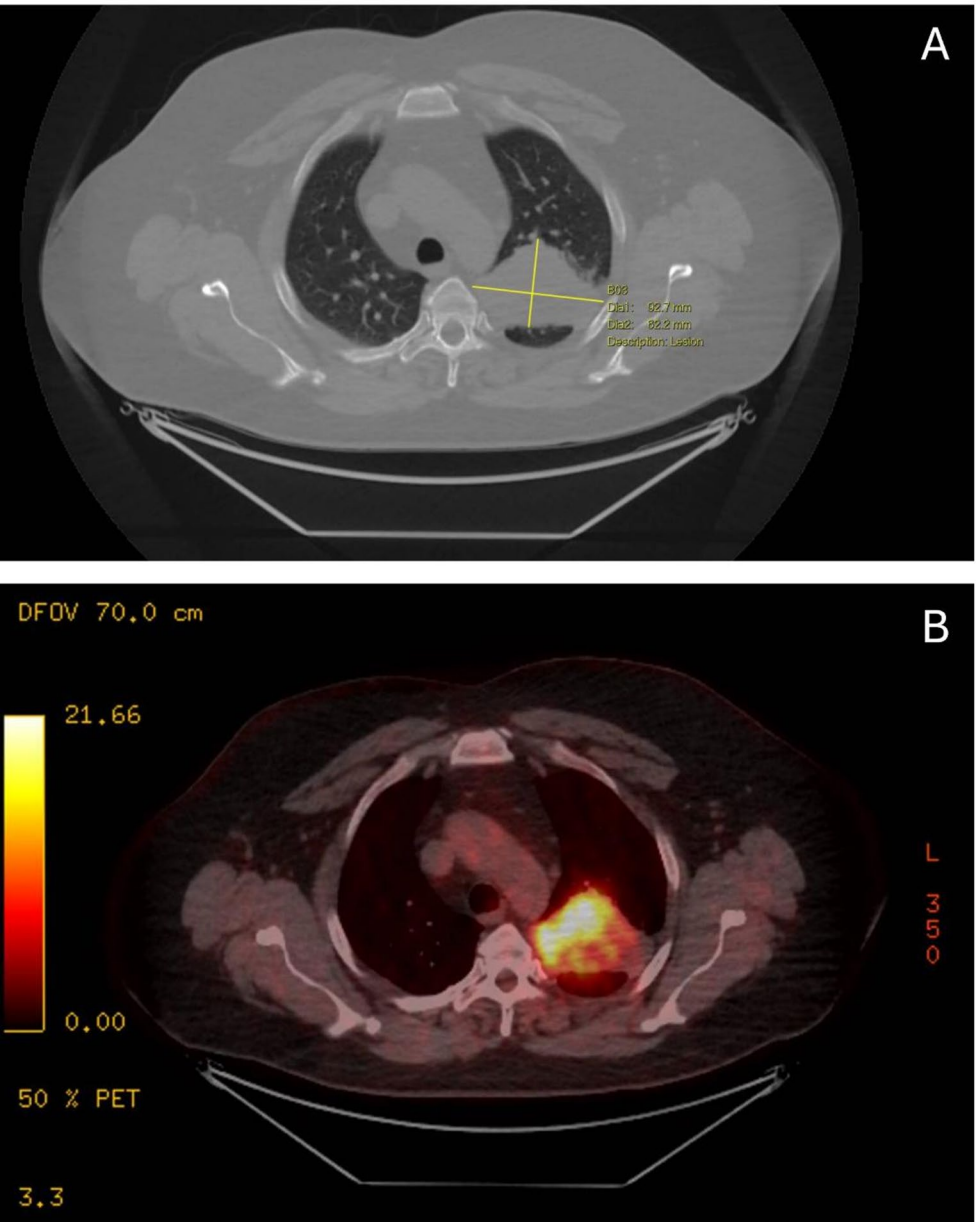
Sectional imaging of the lung lesion on CT A and PET CT B, demonstrating a large PET avid lesion in the apex of the right lung
